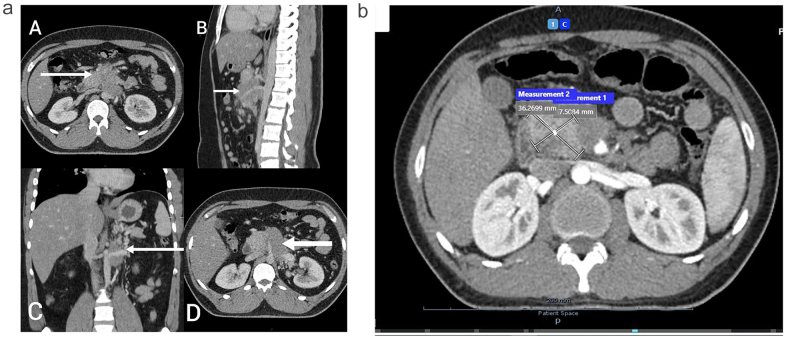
(A) Computed tomographic scan of the abdomen showing heterogeneously enhancing soft tissue density mass in the retroperitoneum. Axial views show the mass abutting the superior mesenteric vein anterolaterally (A) and inferior vena cava posteriorly (D). Lateral view showing the mass encasing the superior mesenteric artery, loss of fat planes, and inferior vena cava posteriorly (B). Frontal view showing the mass encasing the left renal vein (C). (B) Computed tomographic scan of the abdomen and pelvis showing infiltrative hypo-enhancing mass (measuring 8.1 cm in maximum oblique dimension) centered in the retroperitoneum involving the head and uncinate process of the pancreas.
